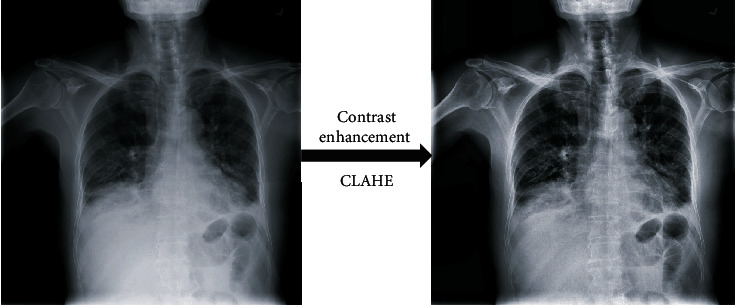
Chest X-ray image preprocessing.
